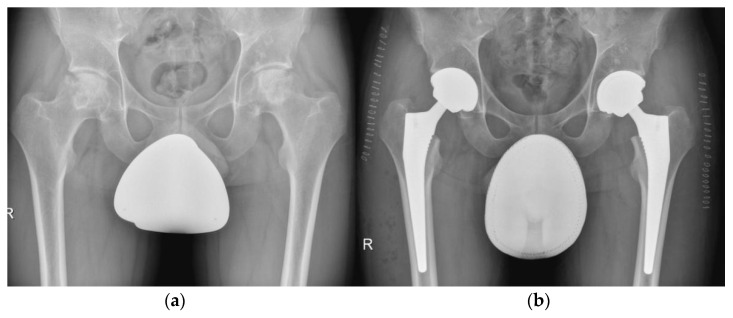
Bilateral total hip arthroplasty (THA). (a) preoperative X-ray; (b) immediate postoperative X-ray after one-stage bilateral total hip arthroplasty.
